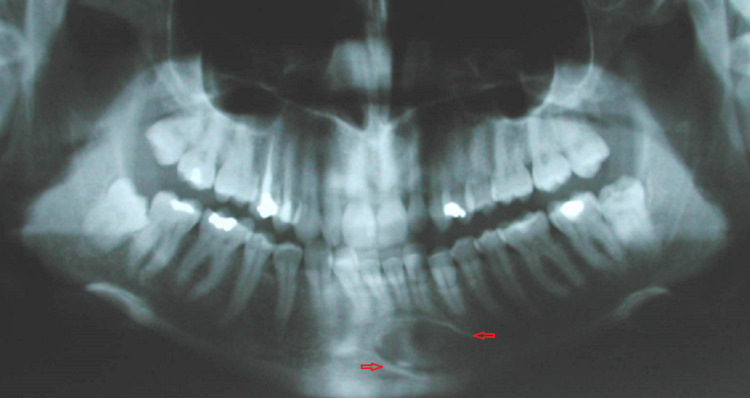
Panoramic radiograph showing radiolucency located in the anterior region of the mandible below the teeth apices
